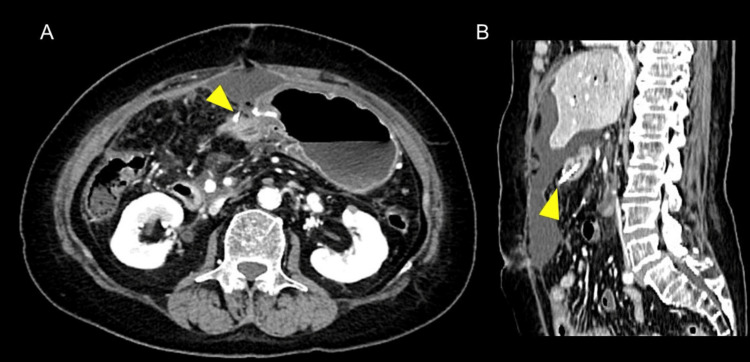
Enhanced abdominal computed tomography of Case 1. Computed tomography showed the pancreatic stent penetrating the jejunum (arrowhead). A: axial view, B: sagittal view.
